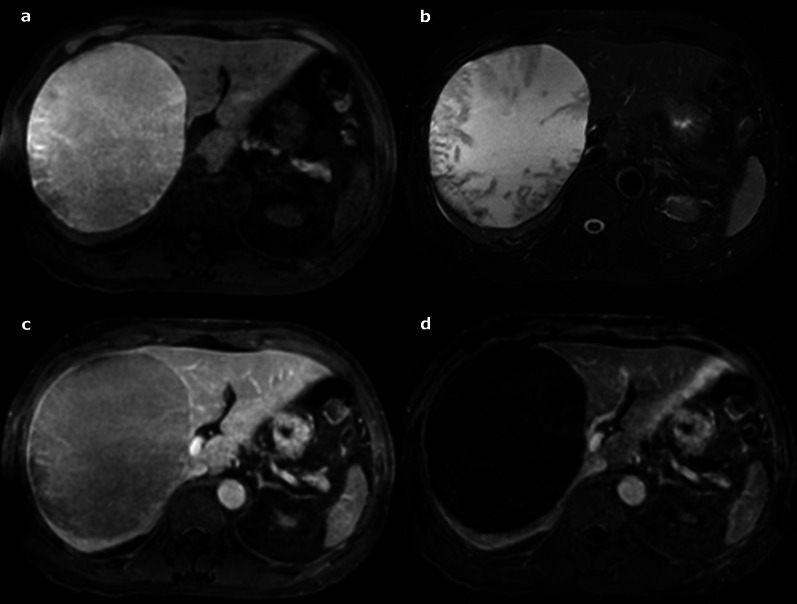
Haemorrhagic cyst in a 55-year-old male patient. a Axial T1 fat-sat-weighted magnetic resonance imaging shows hyperintense lesion and (b) axial T2-weighted magnetic resonance imaging shows a heterogeneous hyperintensity; c and d axial T1-fat-sat-weighted imaging without and with subtraction shows no enhancement after gadolinium-chelate injection
